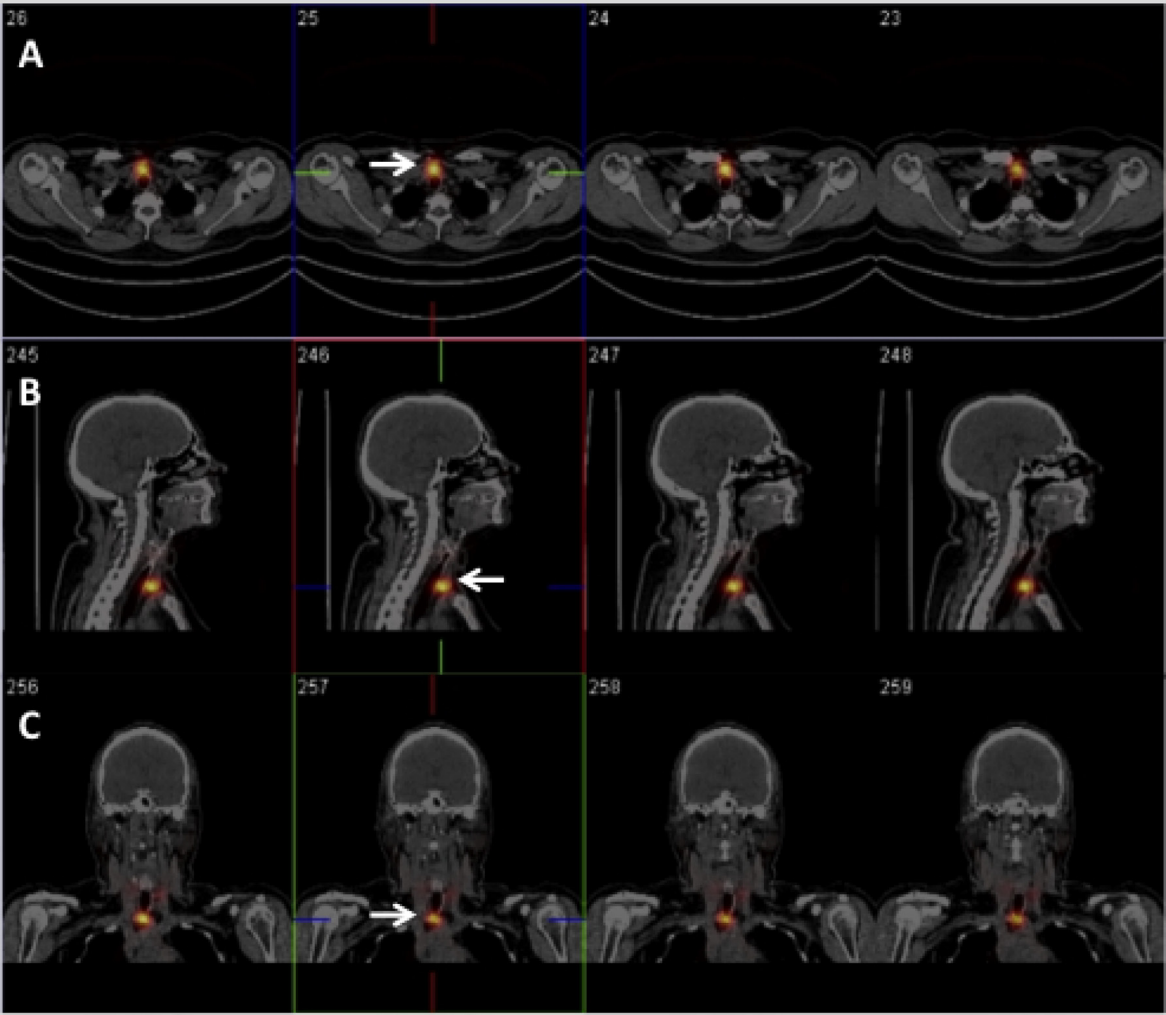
Fifty-eight-year-old woman, pT1aNxMx-PTC (follicular variant) located in the isthmus Patient underwent TRA after 5-weeks L-thyroxine withdrawal. RAIU: 2.5%. Five days after TRA (2753 MBq), axial (A), sagittal (B) and coronal (C) SPECT/CT images identified a focal area of quite intense radioiodine uptake corresponding to central compartment lymph node metastasis (white arrow). At TRA, serum TSH, Tg-Ab and Tg levels were 70 µIU/ml, < 4 ng/ml and 1.0 ng/ml, respectively. Lymph-node metastasis was also confirmed by targeted nUS (10 mm in size).
